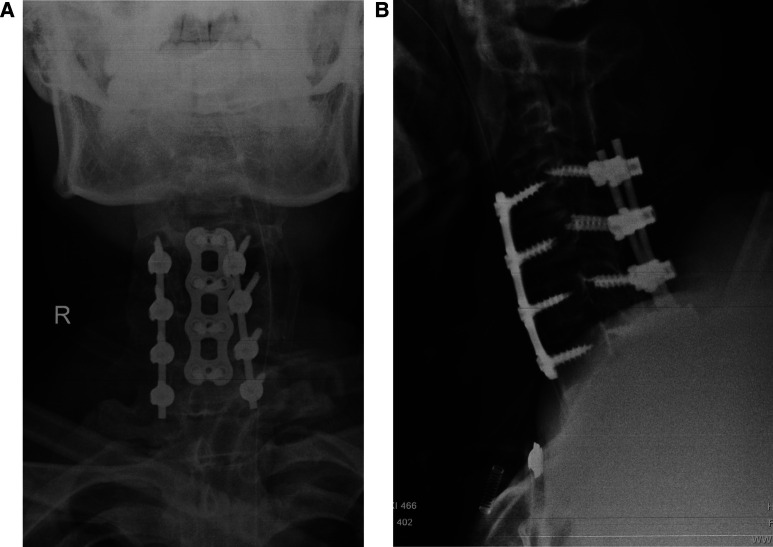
Postoperative plain radiography (A,B).
